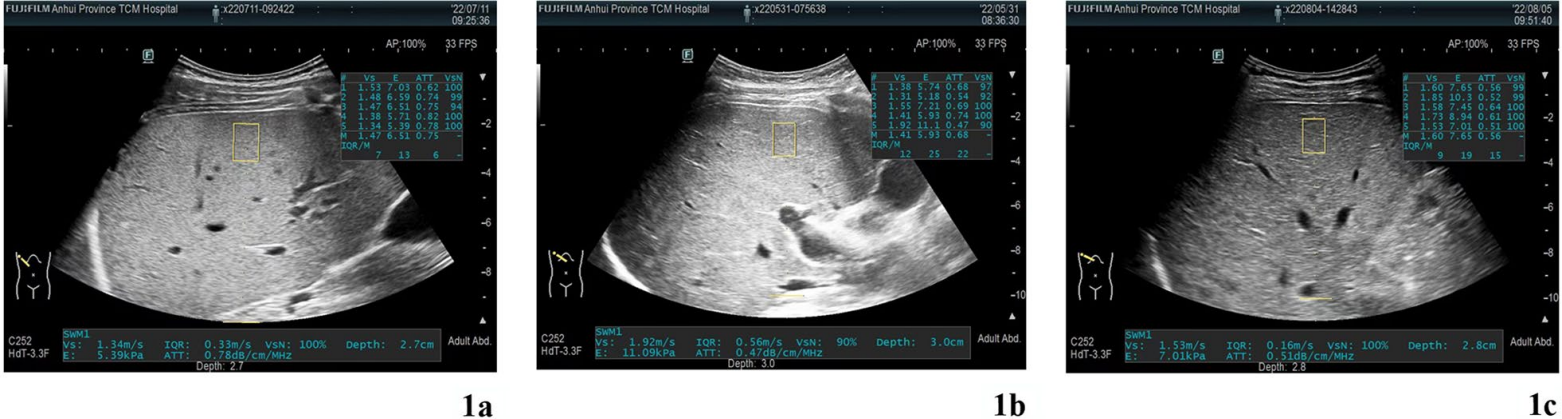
ATT ultrasound images for 3 groups of patients with WD (a Group1, median of ATT is 0.75; b Group1, median of ATT is 0.68, c Group1, median of ATT is 0.56)
